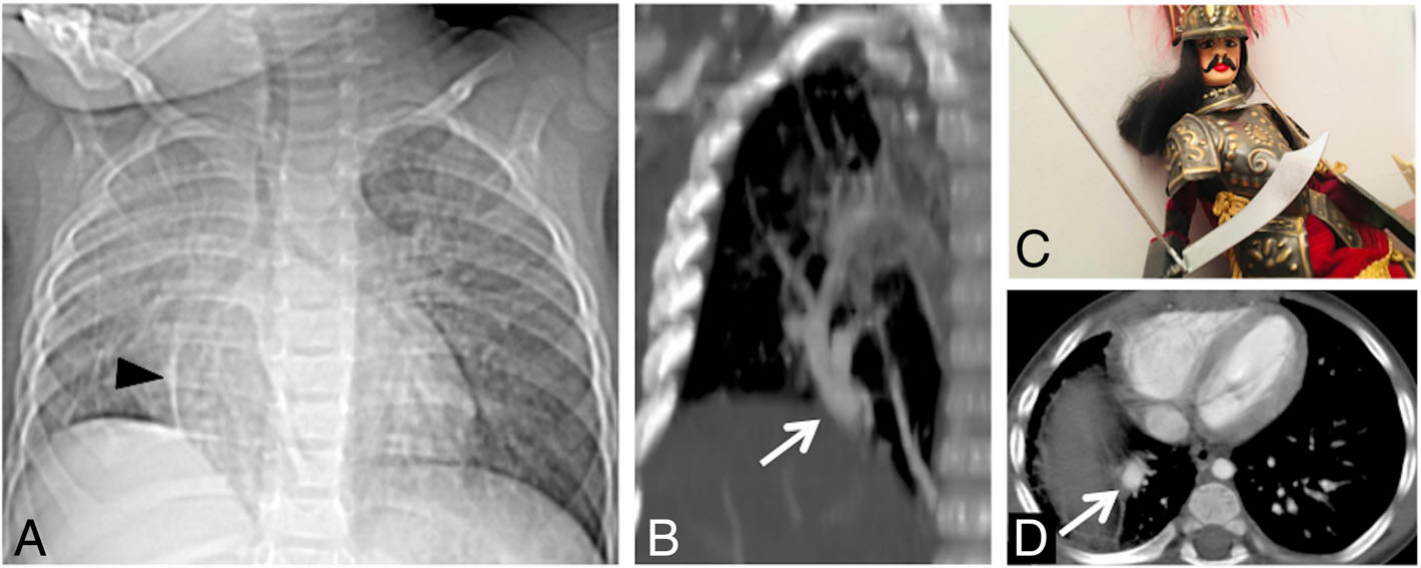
Scimitar sign. Scimitar syndrome in patients with abnormal pulmonary venous return. The scimitar sign (white arrows in a and b) can be easily recognized: the anomalous vessel often runs as a radiopaque tubular structure parallel to the right margin of the cardiac shadow (anterior-posterior CT scout and coronal CT reformatted images), reproducing the shape of the famous Turkish curved sword (c). On axial CT scan (d), the abnormal pulmonary vein is clearly recognizable (white arrow)
